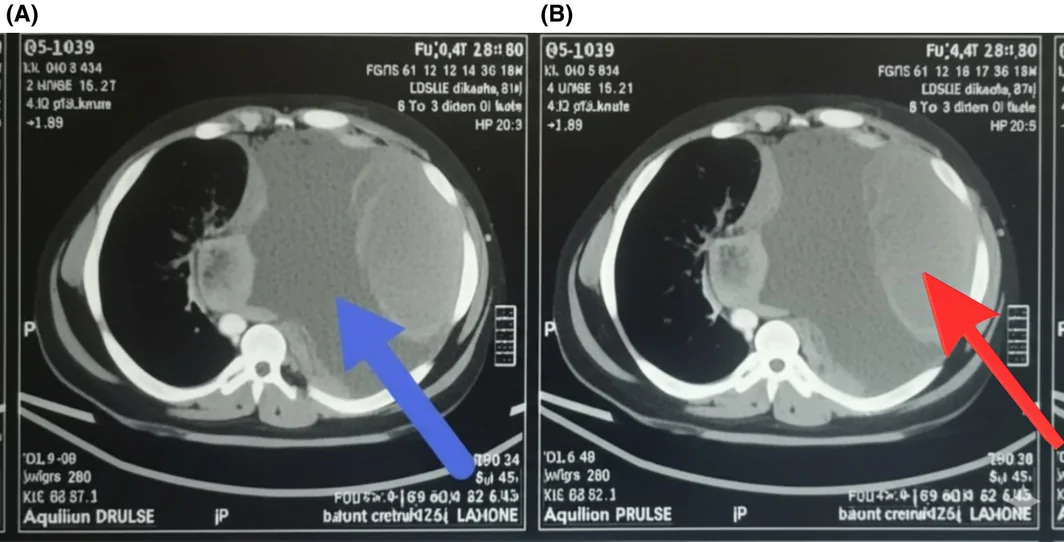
CT scan of the chest showing the soft tissue mass originating from the left rib and pleural fluid. (A) The blue arrow shows pleural fluid. (B) The red arrow shows a soft tissue density lesion in the periphery of the lung.
